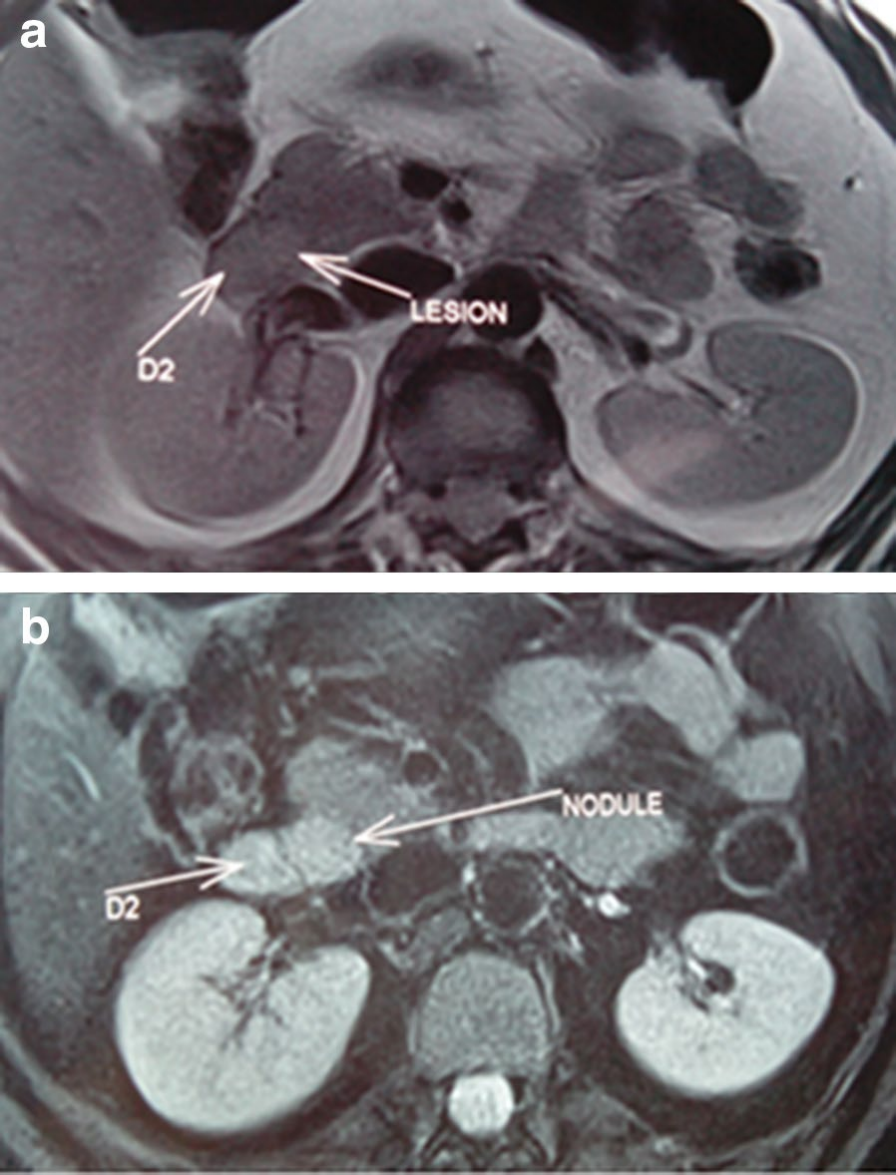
Abdominal magnetic resonance imaging findings: pancreatic nodule with low signal intensity on T1-weighted images (*lesion arrow*) (a) and high signal intensity on T2-weighted images (*nodule arrow*) (b)
